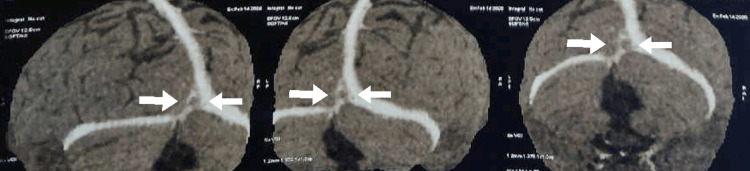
CT venography showing straight sinus and lateral sinuses CT, computed tomography
